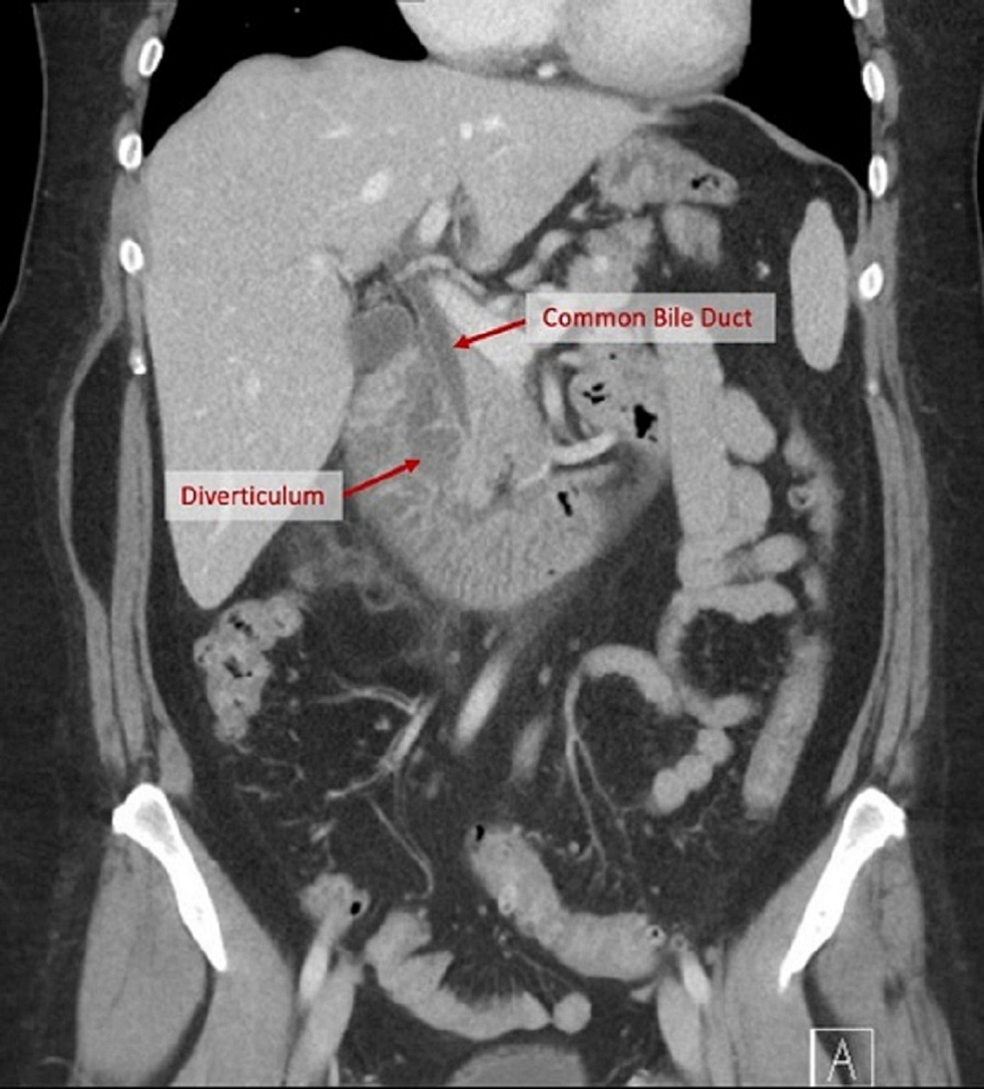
CT abdomen and pelvis showing the common bile duct (CBD) and duodenal diverticulum
